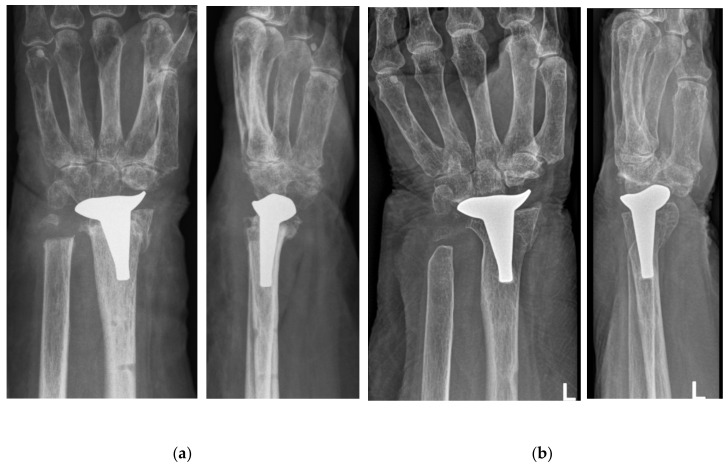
X-rays in posterior-anterior (left) and lateral (right) view 1 day after WHA (a). The follow-up X-ray after 6.5 years showed no sign of implant loosening, subsidence of carpal collapse (b).
